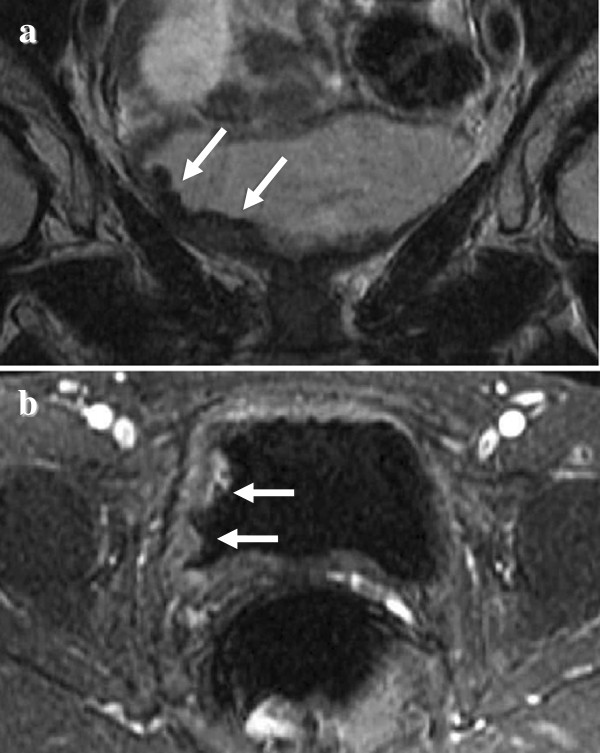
Magnetic resonance imaging findings of the pelvis (a: coronal section, b: transverse section). Magnetic resonance imaging indicated thickness of the right lateral wall of the urinary bladder (arrow).
